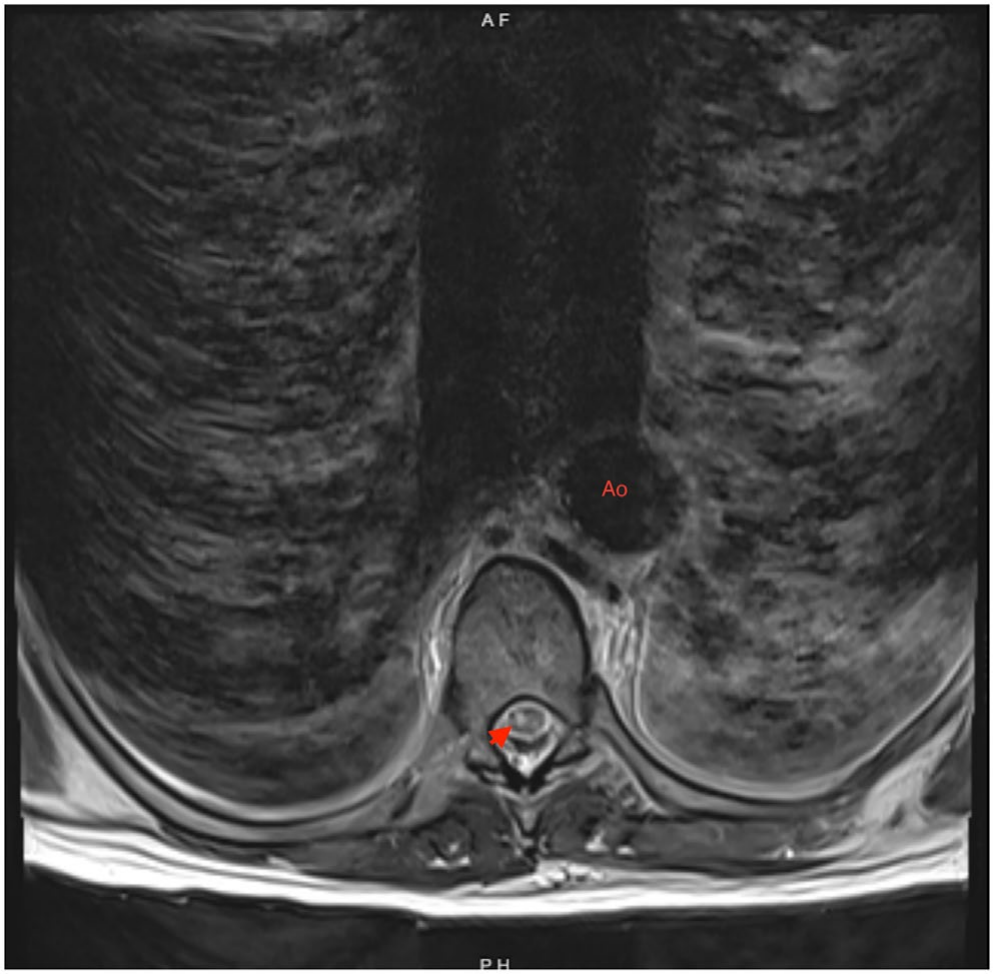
MRI T2 axial view of thoracic spinal cord. There is abnormal T2 hyperintensity (red arrow) within the spinal cord suggestive of cord infarction. Note the aorta with patent endovascular graft (Ao).
